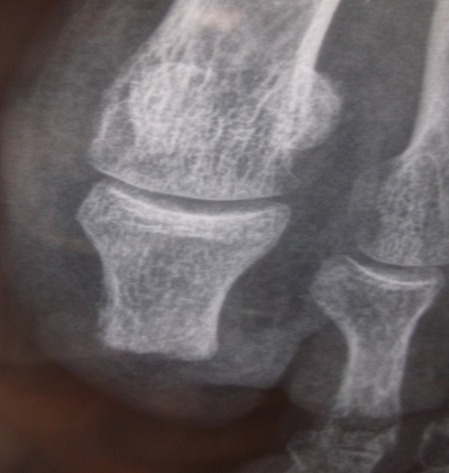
Radiographie de contrôle du moignon d'orteil en post opératoire au septième jour
